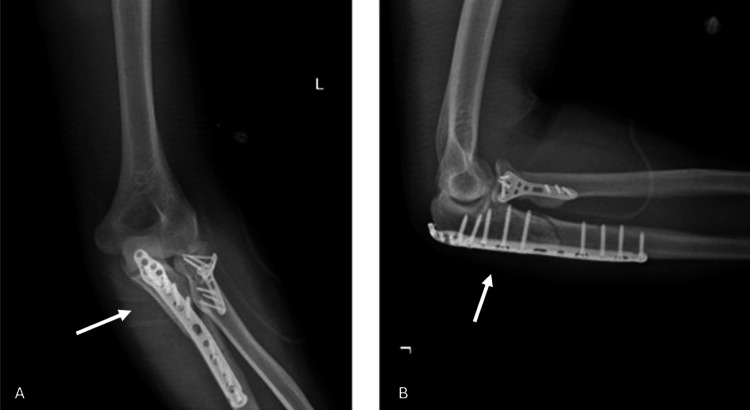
Postoperative X-ray images of the patient can be seen on the anteroposterior (A) and the lateral (B) view White arrows indicate the implant after surgery.
